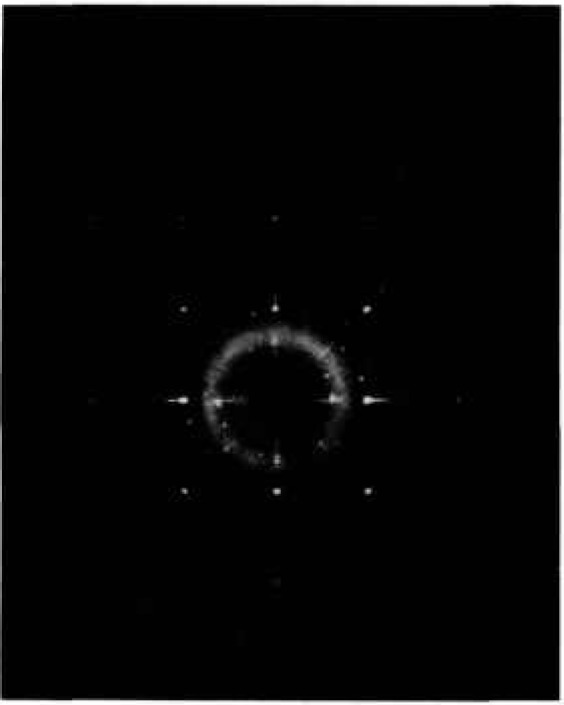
x-ray precession photograph of the bcc distorted phase (Mo radiation).
